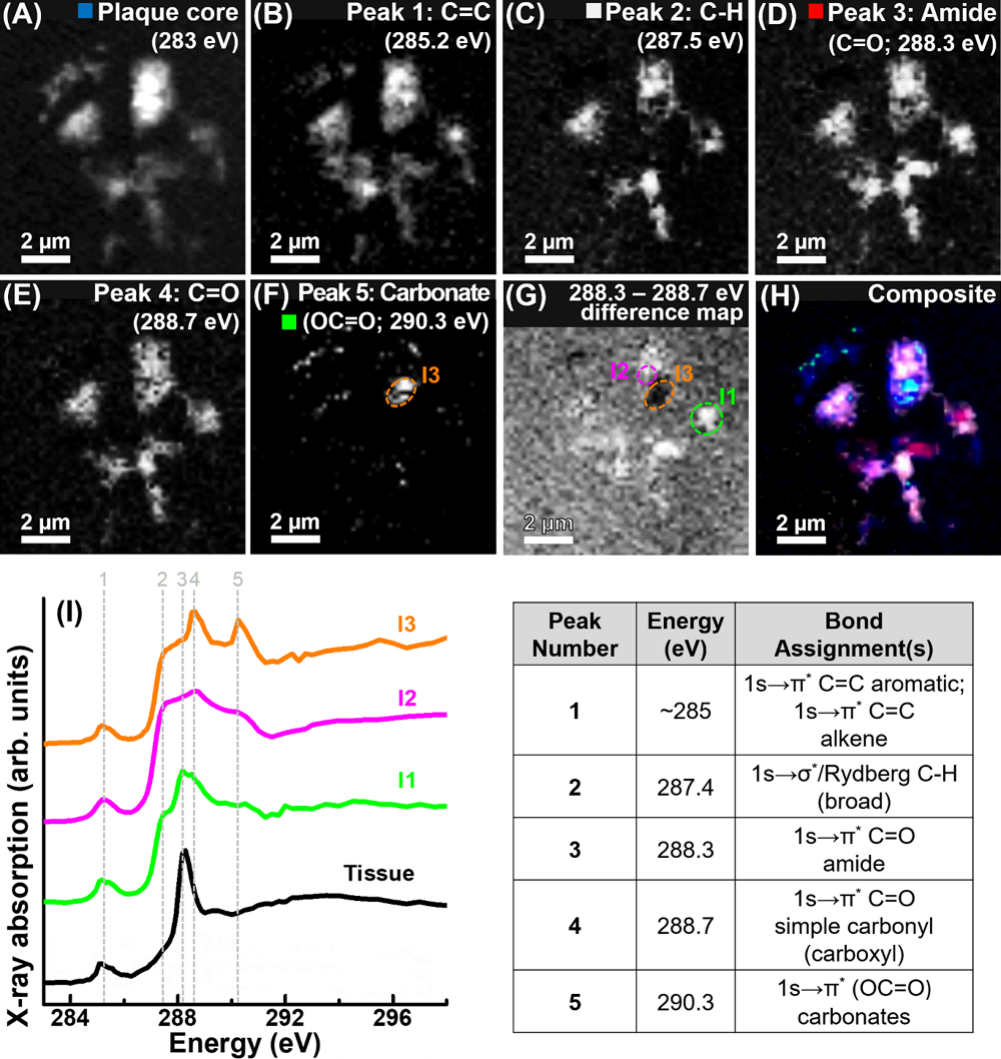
Carbon *K*-edge X-ray images speciation
